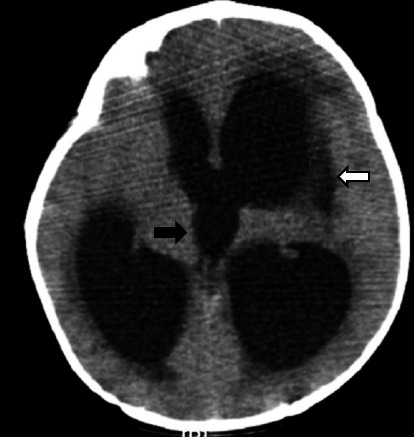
Axial brain CT during the first admission. Demonstrating moderate hydrocephalus (black arrow), with interstitial edema and encephalomalacia (destruction of brain tissue) in the left lentiform nucleus (white arrow).
